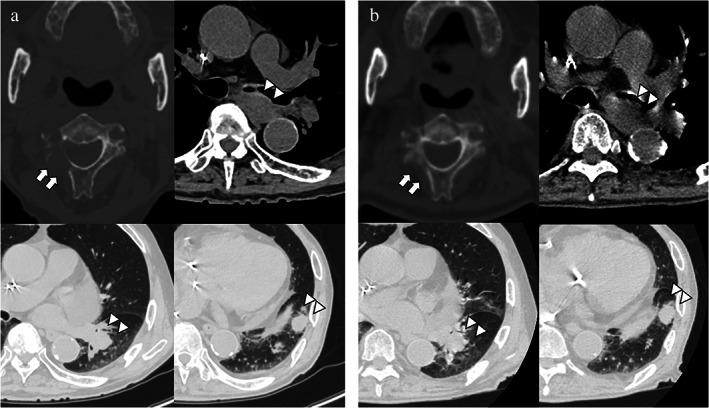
Abscopal effect for only a single lesion. (a) Computed tomography (CT) findings before radiation therapy to the right iliac bone metastasis. (b) CT findings after the radiation therapy. The white arrows show abscopal effect of axial vertebral bone metastasis and white arrowheads indicate other tumor lesions without change in tumor size
